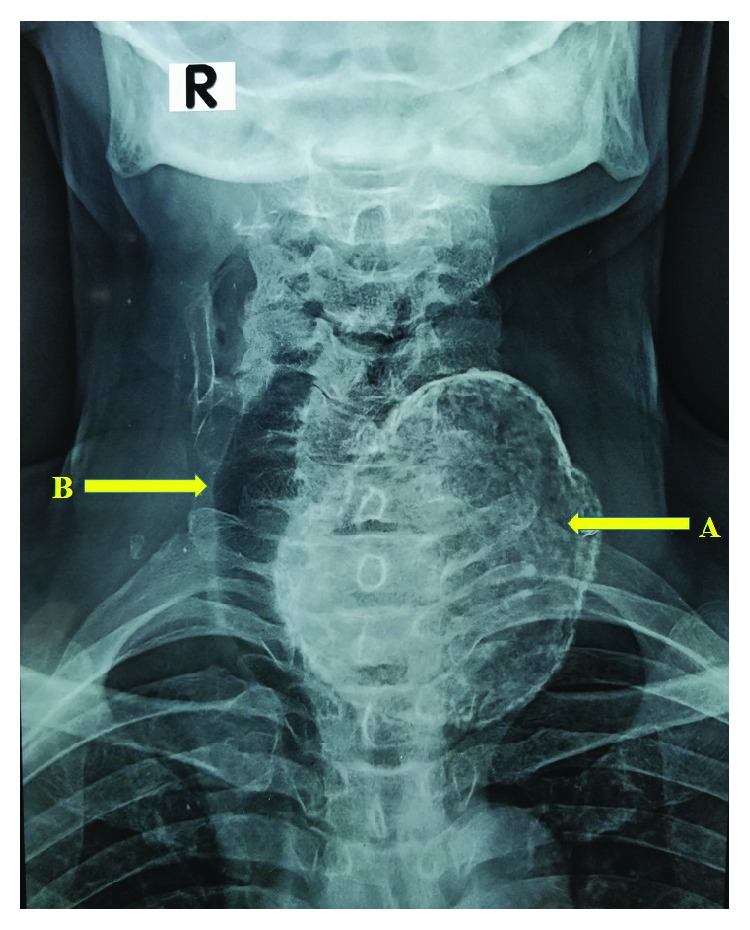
Neck X-ray radiography (anteroposterior view) showing the calcified left lobe (arrow A) with significant tracheal deviation to the opposite side (arrow B).
